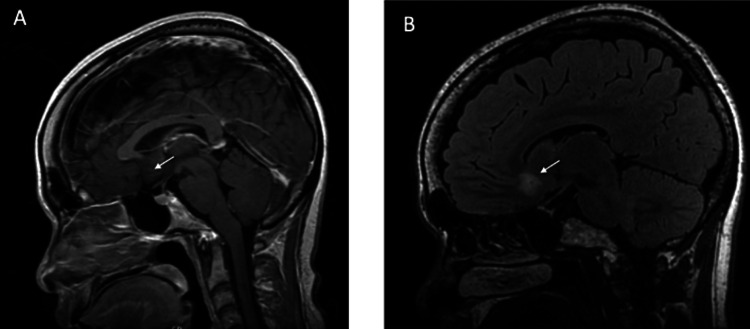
Subject 2 MRI brain at (A) 12 and (B) 32 months post-SRS The white arrows show the area of lesioning in the subgenual cingulate. SRS, stereotactic radiosurgery
